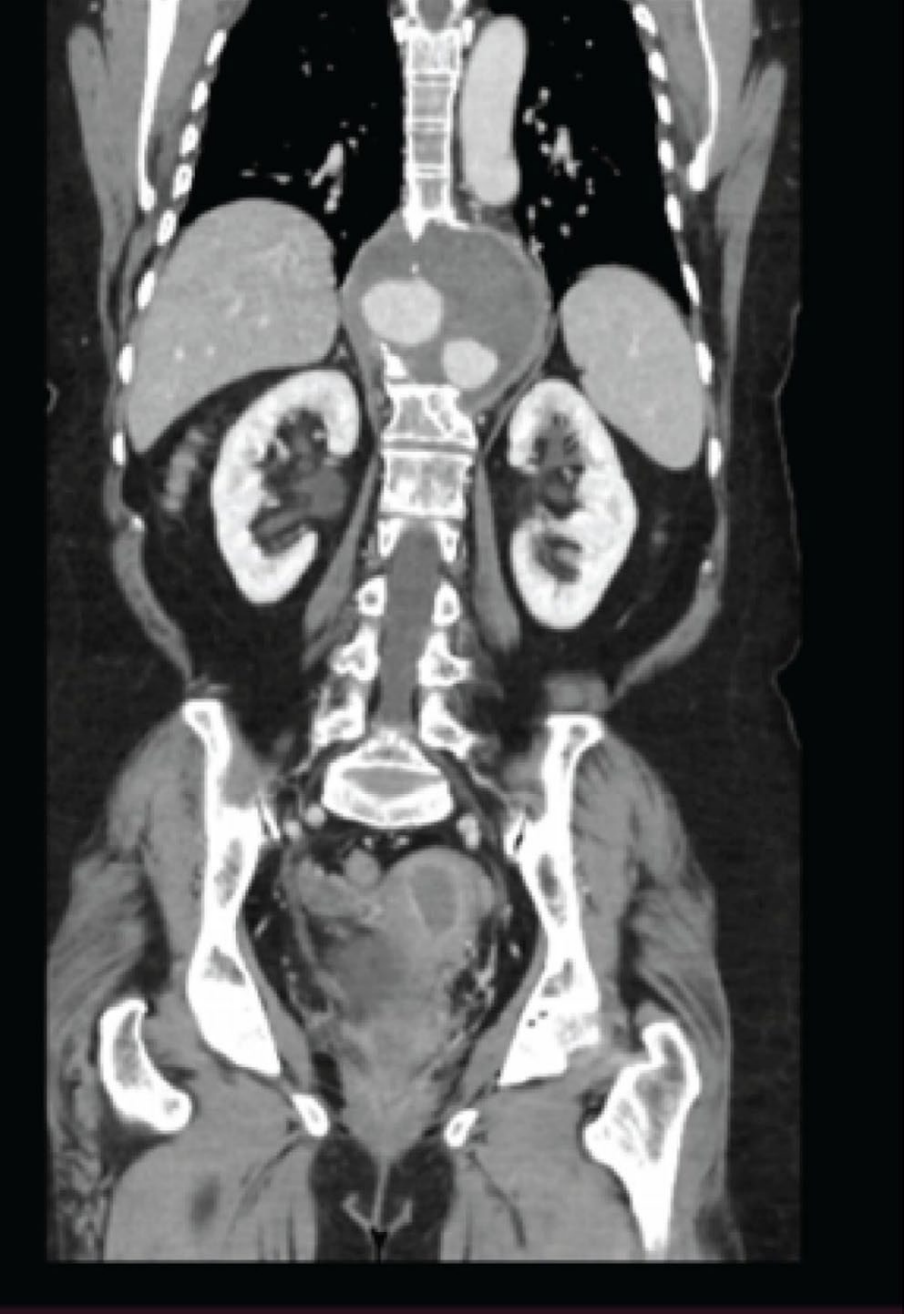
Coronal CT angiographic view showing asymmetrical involvement of the vertebral bodies. Erosion is more pronounced on the left side, correlating with the left‐sided origin of the aneurysm bulge.
